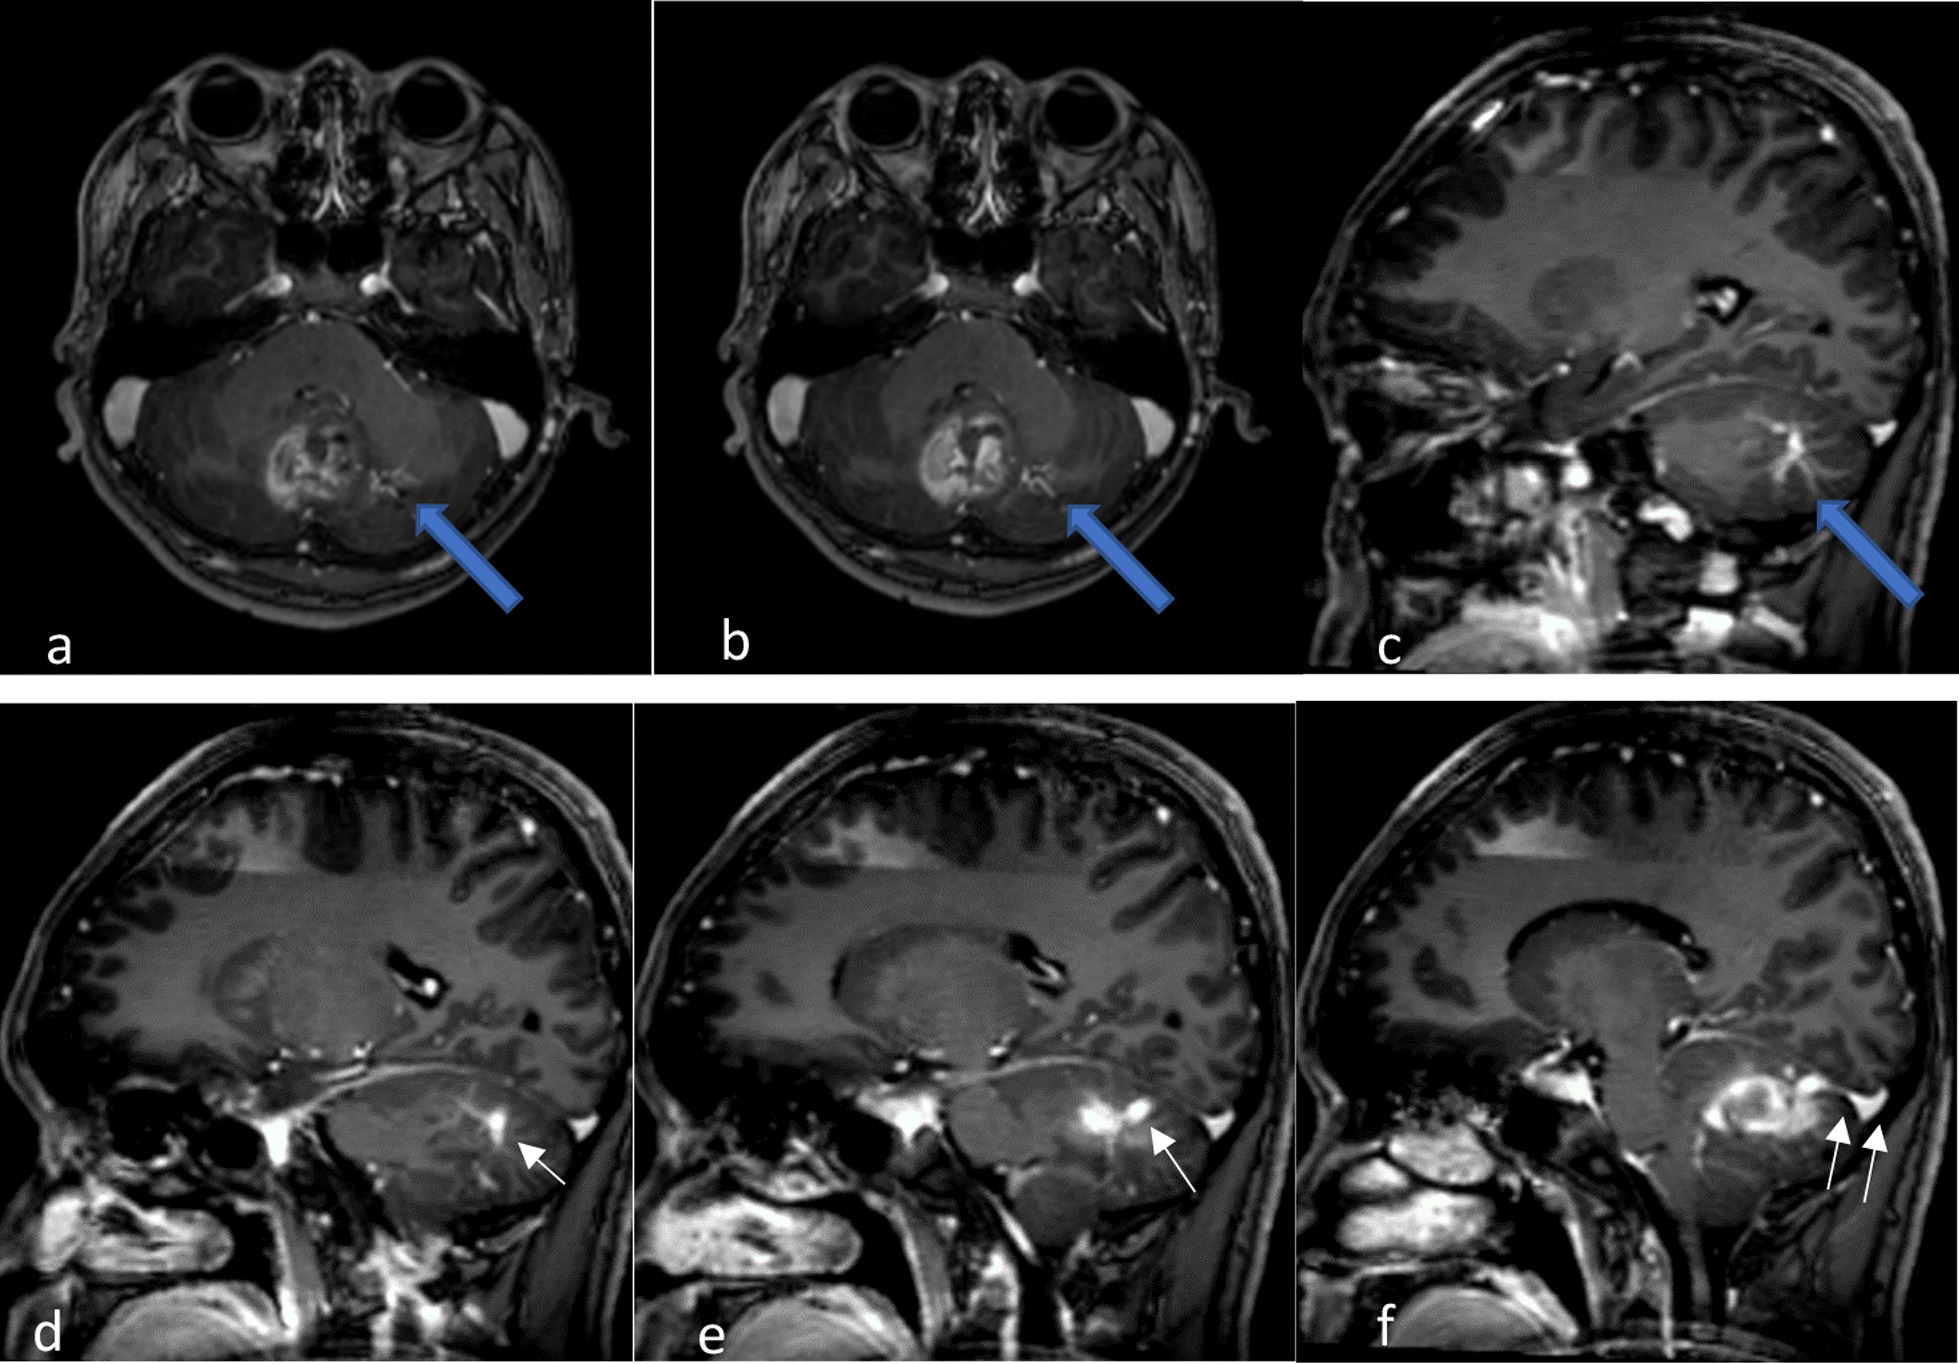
Axial post-contrast MRI (a, b) and sagittal MRI (c) showing enhancing tubular structure at left cerebellum resembling caput medusa sign (blue arrow in a–c) in keeping with developmental venous anomalies (DVAs). Note that the veins drain centrally toward a single draining vein (white arrow in d–f), draining into the torcular Herophili
